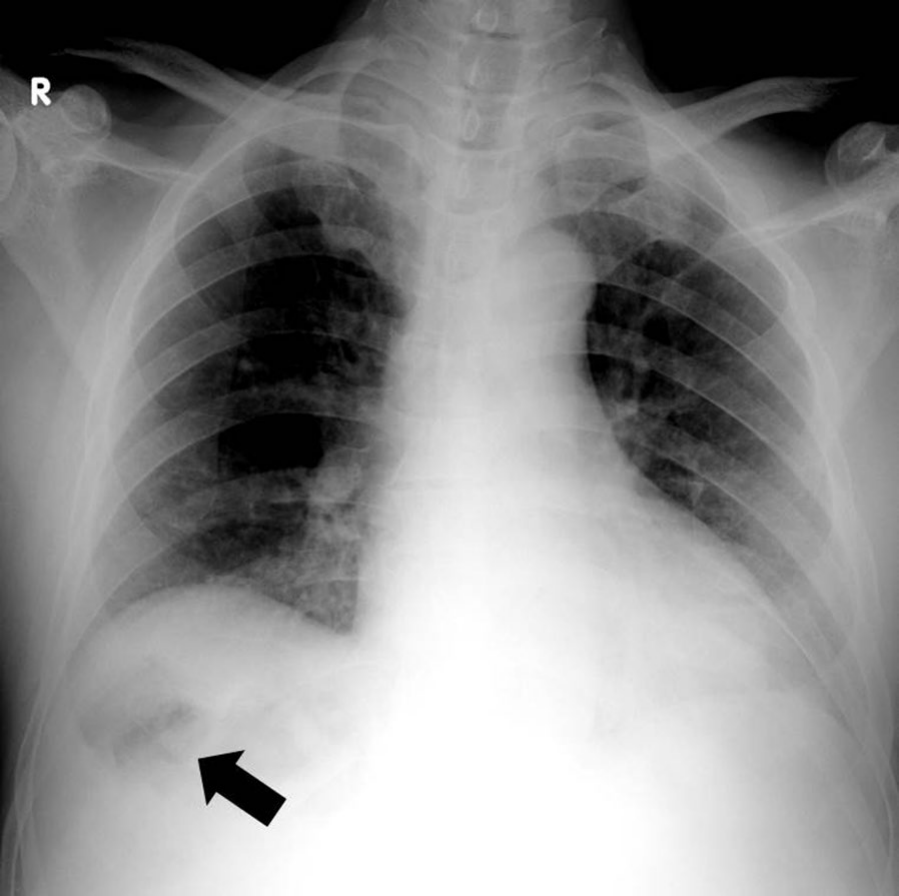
Gas was observed in the right subphrenic space on simple abdominal radiography (*arrow*)
